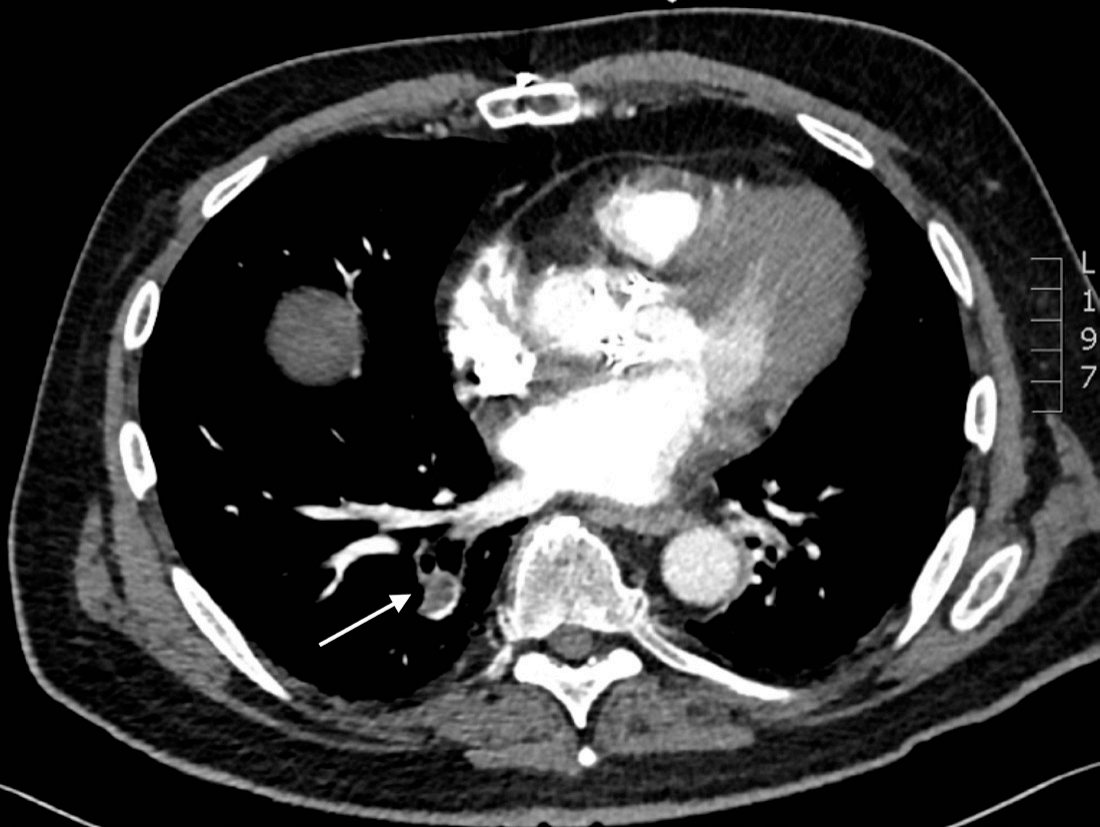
Computed tomography of the chest with contrast demonstrating a right lower lobe pulmonary embolism (arrow).
